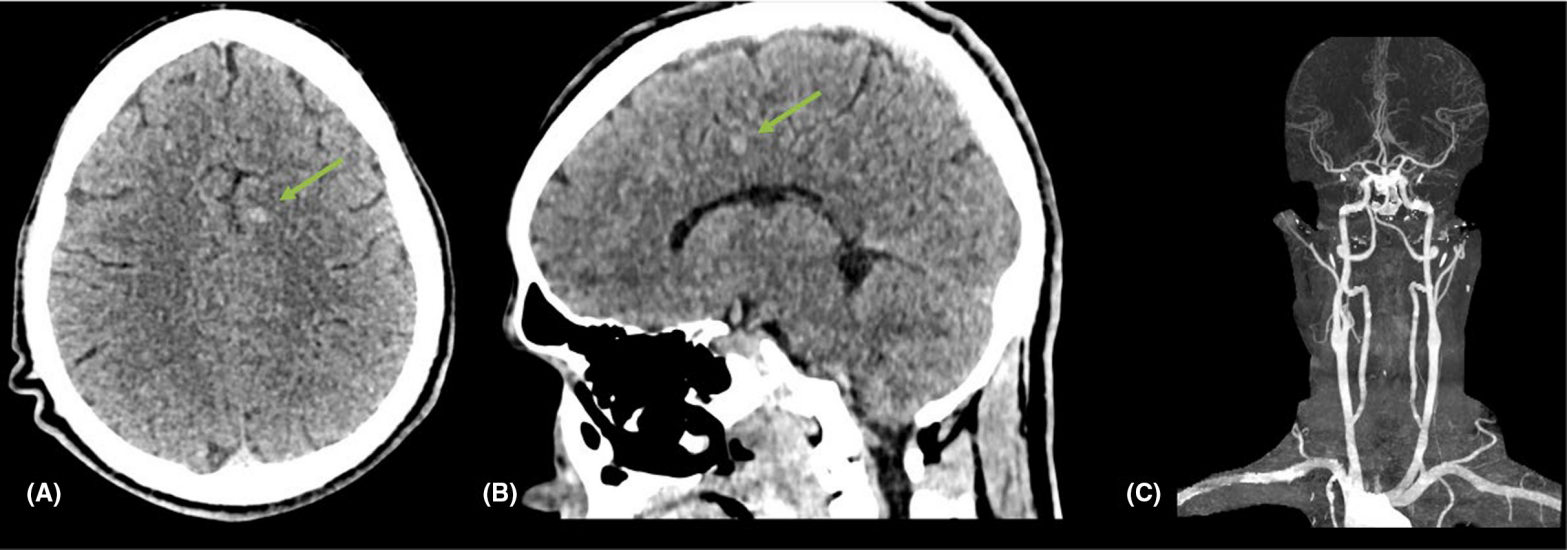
Non‐contrast CT head: (A) Axial and (B) Sagittal selected cuts, showed a hyperdense focus measuring 4 × 6 mm devoid of perilesional oedema in the left parasagittal frontal lobe region (green arrows) suggestive of a cavernoma or a petechial hemorrhage. (C) A CT intracranial angiogram was unremarkable.
